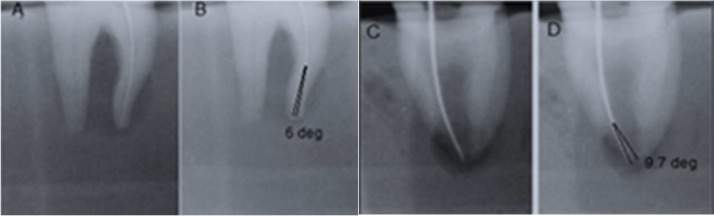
Representative example of radiographs taken in *A)* Buccolingual, before preparation; *B)* Mesiodistal direction after superimposition; *C)* Buccolingual before preparation and *D)* Mesiodistal after superimposition. The root canal curvature was measured prior to instrumentation with the initial instrument inserted (File size ≠15
